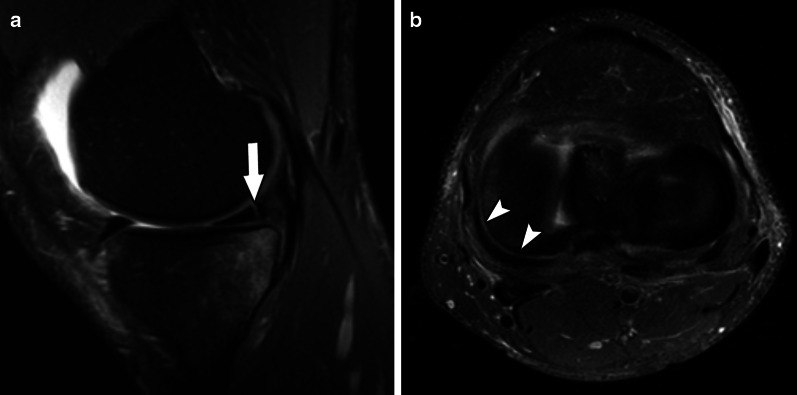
Type 4A meniscal ramp lesion MRI Case. Sagittal (a) and axial (b) T2-weighted fat-suppressed MR image shows a type IV ramp lesion, with tear extending from femoral to tibial articular surfaces (arrow); tear extension is seen in (b) (arrowheads)
